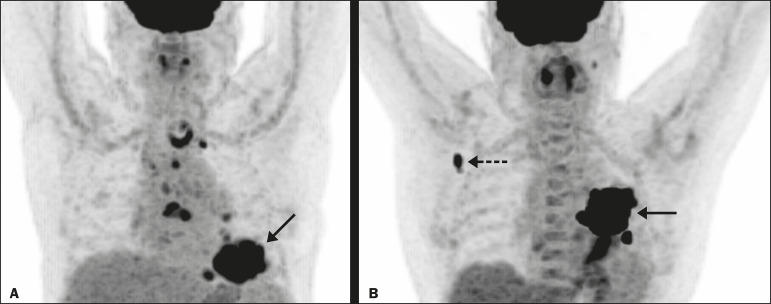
Examples of the importance of the tumor burden, calculated from ^18^F-FDG PET/CT imaging, as a prognostic indicator. A: A patient with stage IIIB NSCLC. The primary tumor (arrow) shows intense uptake (SUV = 13.2), and the multiple mediastinal lymph node metastases have intense hypermetabolism. B: A patient with stage IV NSCLC. The primary tumor (arrow) shows intense uptake (SUV = 47.2), and the patient was classified as having stage IV disease due to a contralateral axillary lymph node metastasis (dotted arrow). According to the TNM staging, the estimated 5-year OS rate was 19% for the patient depicted in A and 6% for the patient depicted in B. The wbTLG/tuTLG ratio was 1.27 for the patient in A and 1.01 for the patient in B. The patient in A had a higher tumor burden and died 11.57 months after diagnosis, whereas the patient in B, despite having been classified as having stage IV disease, was alive 25.7 months after diagnosis. Therefore, the wbTLG/tuTLG ratio, which is an independent prognostic indicator, may be a more accurate tool for assessing OS, which is especially useful in oligometastatic NSCLC.
